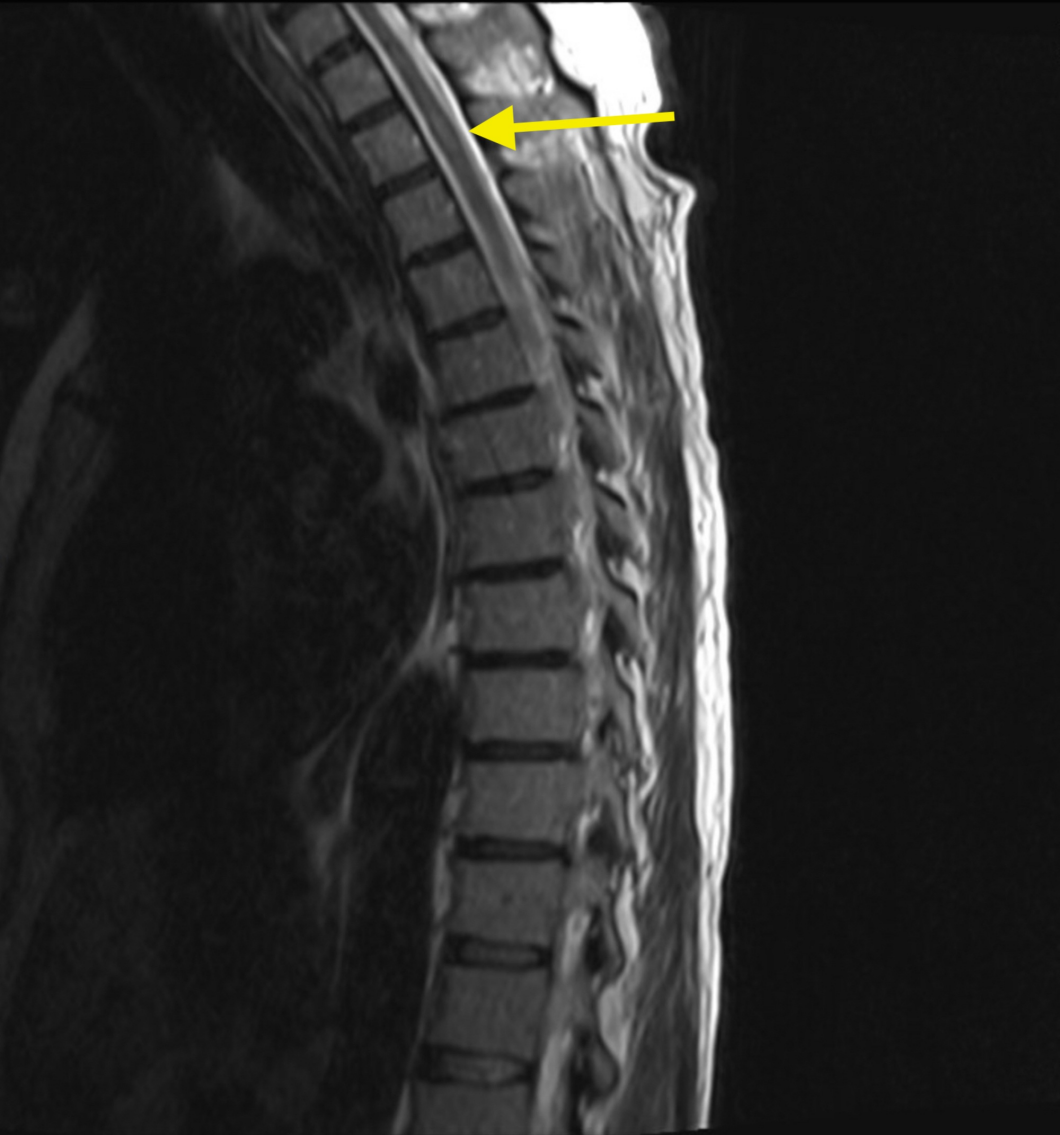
Magnetic resonance imaging (MRI) of the thoracic spine revealing hyperintense lesion at T2 level (yellow arrow).
